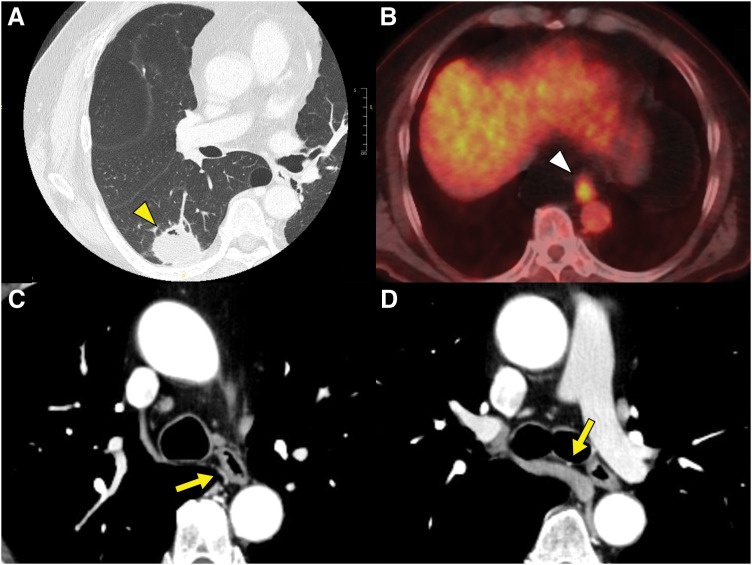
Chest CT revealed a 3.7 cm consolidative mass with irregular margins in the right lower lobe (yellow arrowhead in A). PET demonstrated an abnormal FDG uptake in the lower thoracic esophagus (white arrowhead in B). The right bronchial artery (yellow arrows) originated from the descending aorta, traversed dorsally to the esophagus (C), and passed beneath the azygos arch to supply the right bronchus (D).
